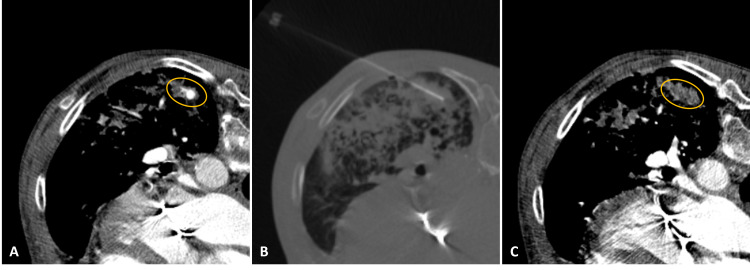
Percutaneous embolization treatment. (A) Post-contrast axial CT image with the patient in the prone position demonstrating the body of the pseudoaneurysm (circle). (B) Same axial CT plane confirming the correct position of the injection needle. (C) Control post-contrast axial CT image showing complete thrombosis of the pseudoaneurysm (circle) after percutaneous injection of thrombin.
